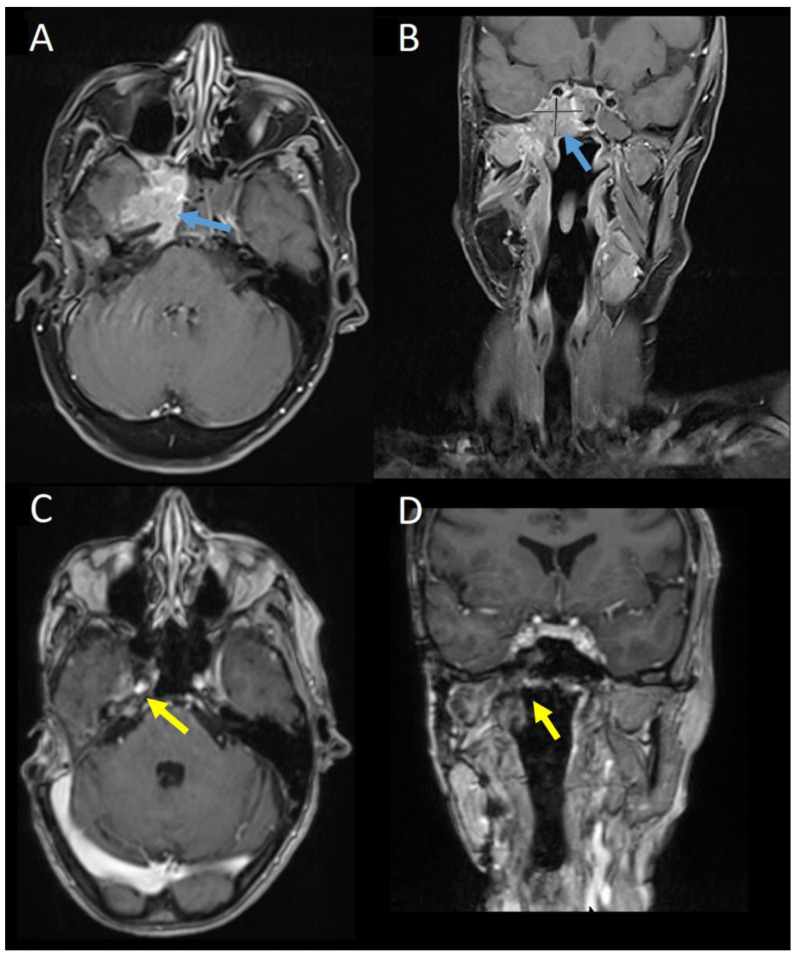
Gadolinium-enhanced MRI scan at baseline in (A) axial and (B) coronal planes and 38 months post-treatment in (C) axial and (D) coronal planes. The enhancing mass centred on the right cavernous sinus in (A,B) (blue arrows) is no longer apparent in (C,D) (yellow arrows).
